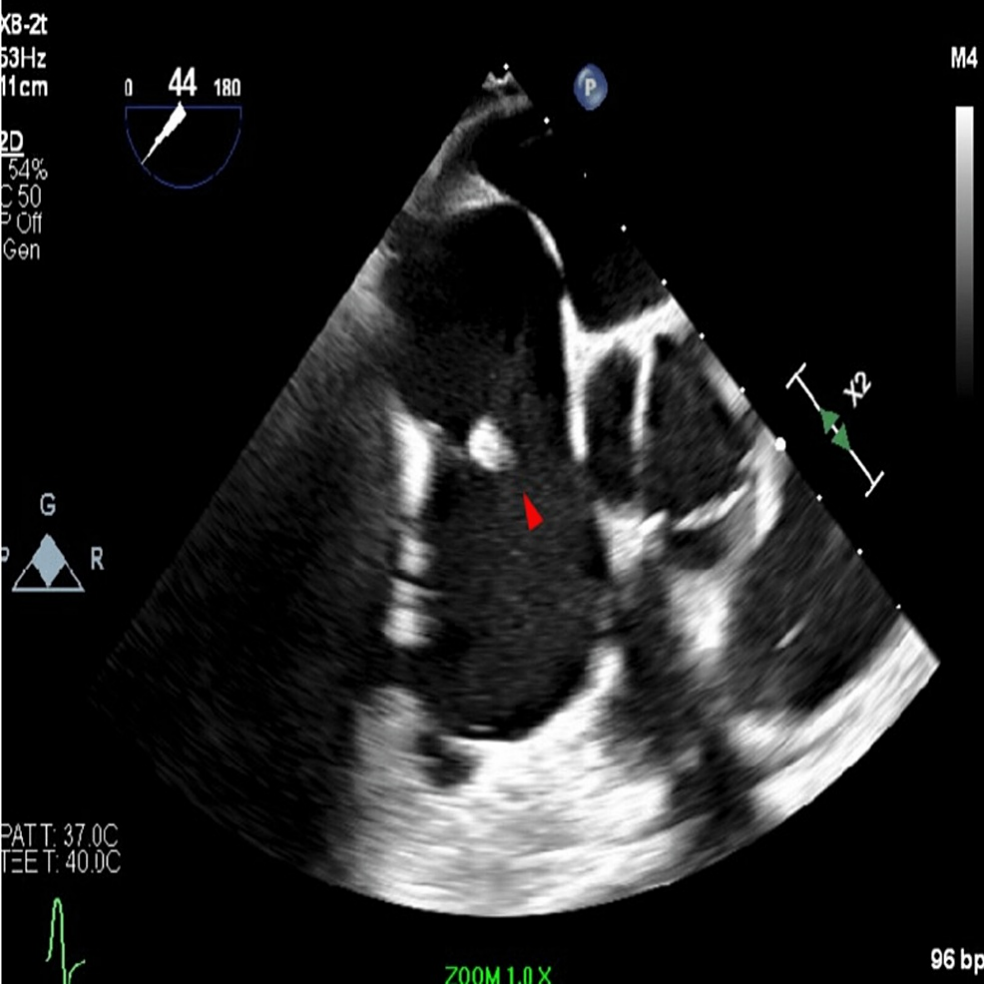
TTE showing a mobile right atrial mass attached to the valve TTE: transthoracic echocardiogram
